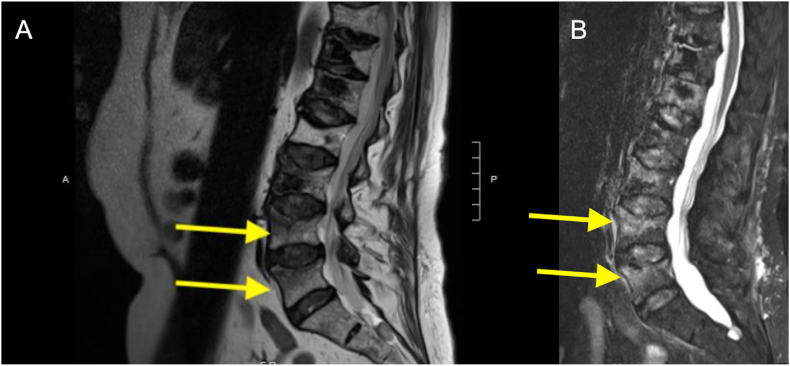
New L4 and L5 12 vertebral compression fractures visualized using MRI sequences. (A) T2-weighted MRI with an arrow pointing to the fracture site. (B) STIR sequence with an arrow highlighting the bright signals indicative of edema/inflammation at the corresponding fracture location.
